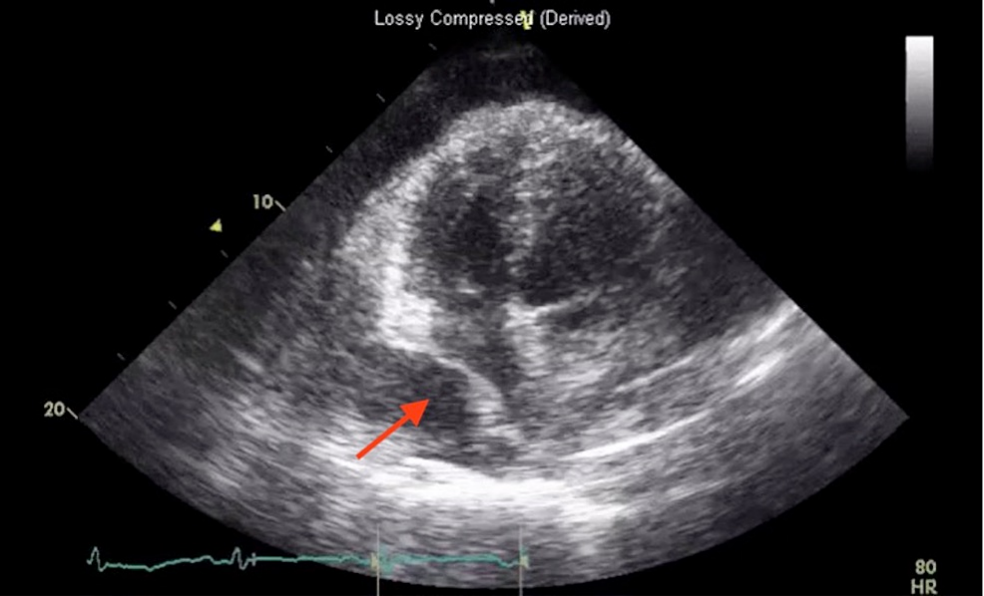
Apical four chambers view of ventricular systolic demonstrating right atrial collapse (arrow)
